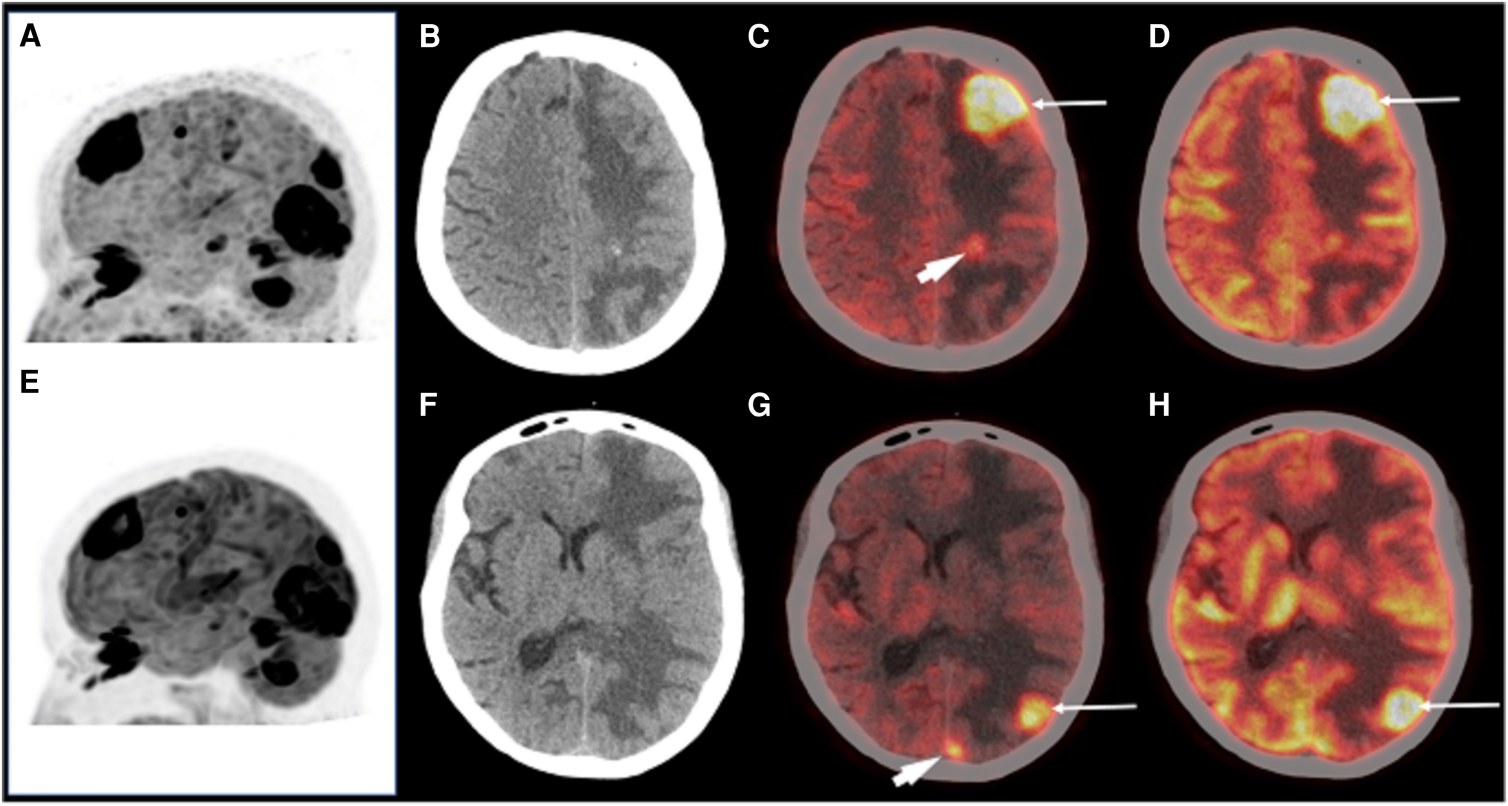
A patient with brain metastases imaged with ^68^Ga-DOTA.SA.FAPi (A,C,G) and ^18^F-FDG (E,D,H) for restaging. MIP images (A,E) showing multiple foci of intense tracer uptake in both. Axial CT revealed ill-defined isodense lesions in the left frontal, parietal, and occipital lobes with perilesional edema (B,F). Images showing increased ^18^F-FDG uptake in these lesions (C,D,G,H arrows). ^68^Ga-DOTA.SA.FAPi also revealed small occult lesions in the left parietal and left occipital lobe (C,G arrowhead). Reproduced with permission from (58).
